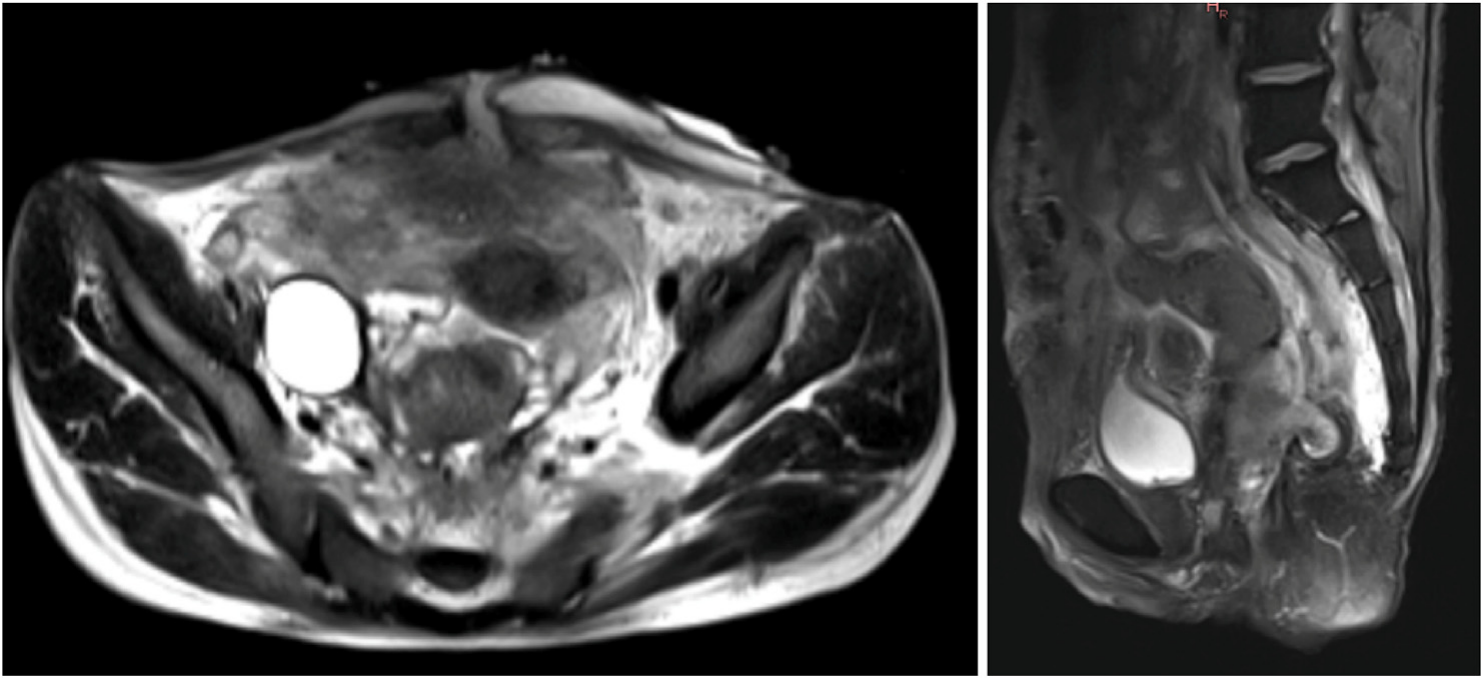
Abdominal MRI - A poorly-dissociable conglomerate of ileal loops was also described, specially involving the pelvic and terminal ileum, with widespread thickening and enhancement of the mesentery, due to phlogosis, and several entero-enteric/entero-mesenteric fistulas.
